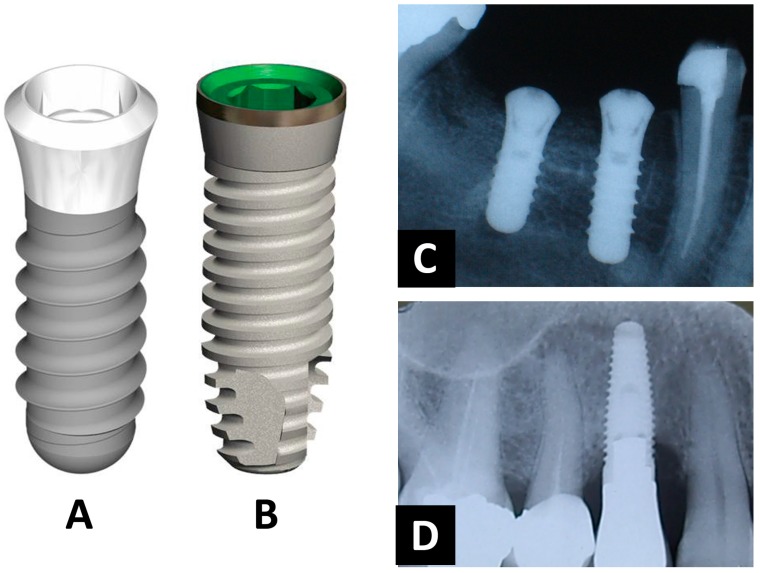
Dental implants are mostly available as (A) threaded cylindrical-shaped or (B) conical (root)-shaped (Images are courtesy of Baltic Osseointegration Academy/Public Information. http://www.boaoffice.lt/EN/9/). Radiographic images displaying clinical cases in which both ((C,D), respectively) implant-designs are inserted in the jaw bone.
